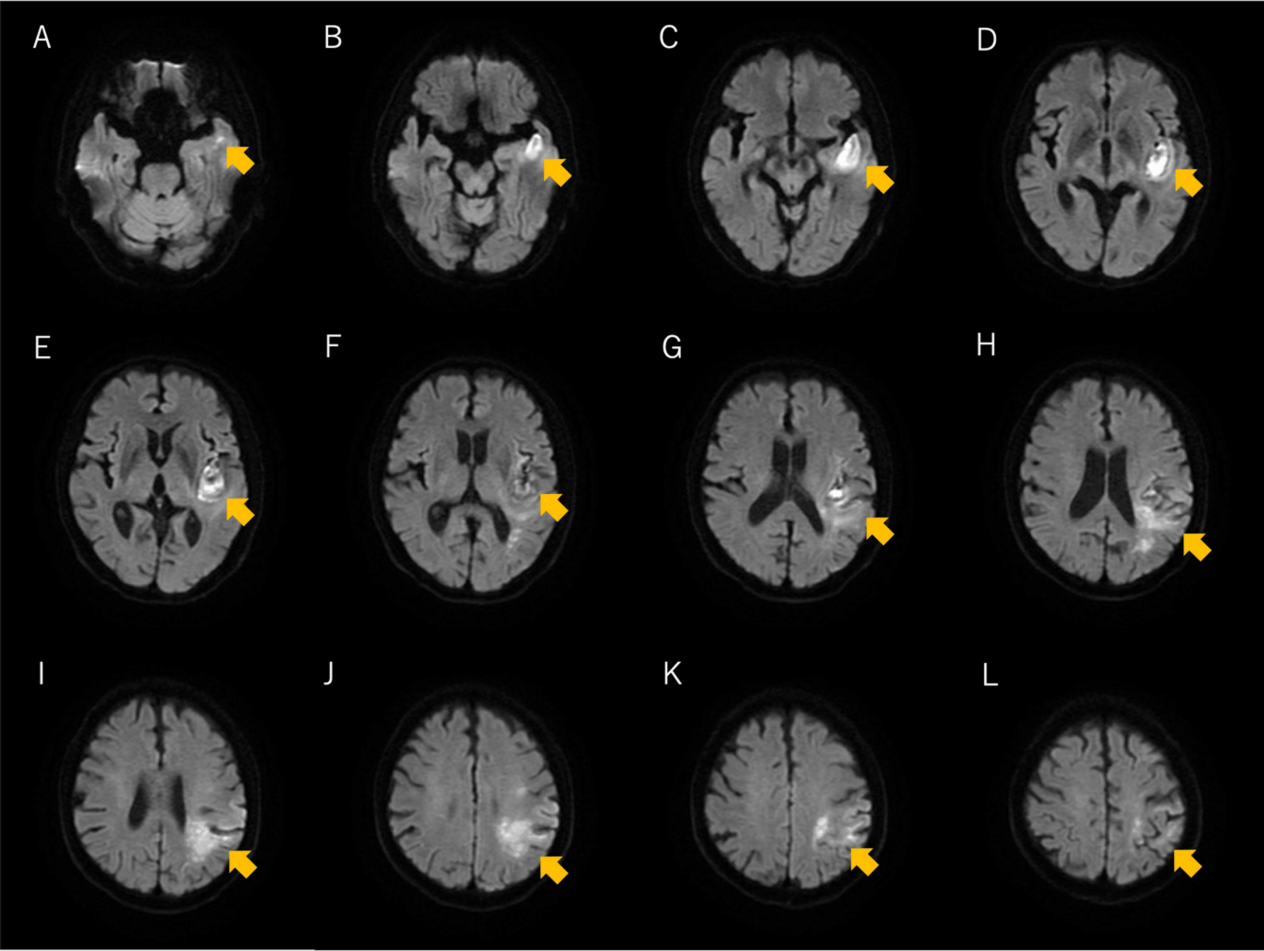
Diffusion-weighted MRI showing acute ischemic stroke lesions Axial DWI on day 12 post-onset reveals hyperintense ischemic lesions (yellow arrows) in the left temporal and parietal lobes. (A-D) Lesions are visible in the posterior superior temporal gyrus (Wernicke's area) and the middle temporal gyrus. (E-H) Involvement extends to the left parietal lobe, specifically affecting the supramarginal and angular gyri, which are linked to praxis and action sequencing. (I-L) Lesions are also observed near the parietal cortex, close to the sensorimotor area, correlating with motor deficits and apraxia symptoms. These findings align with the patient's severe receptive aphasia, apraxia, and motor planning impairments. An MRI was taken on the second day of illness, but the image quality was poor, and the MRI on the 12th day clearly showed an infarct lesion in the parietal lobe and an infarction in the temporal lobe. Therefore, I posted a 12-day MRI scan. MRI: magnetic resonance imaging; DWI: diffusion-weighted imaging
